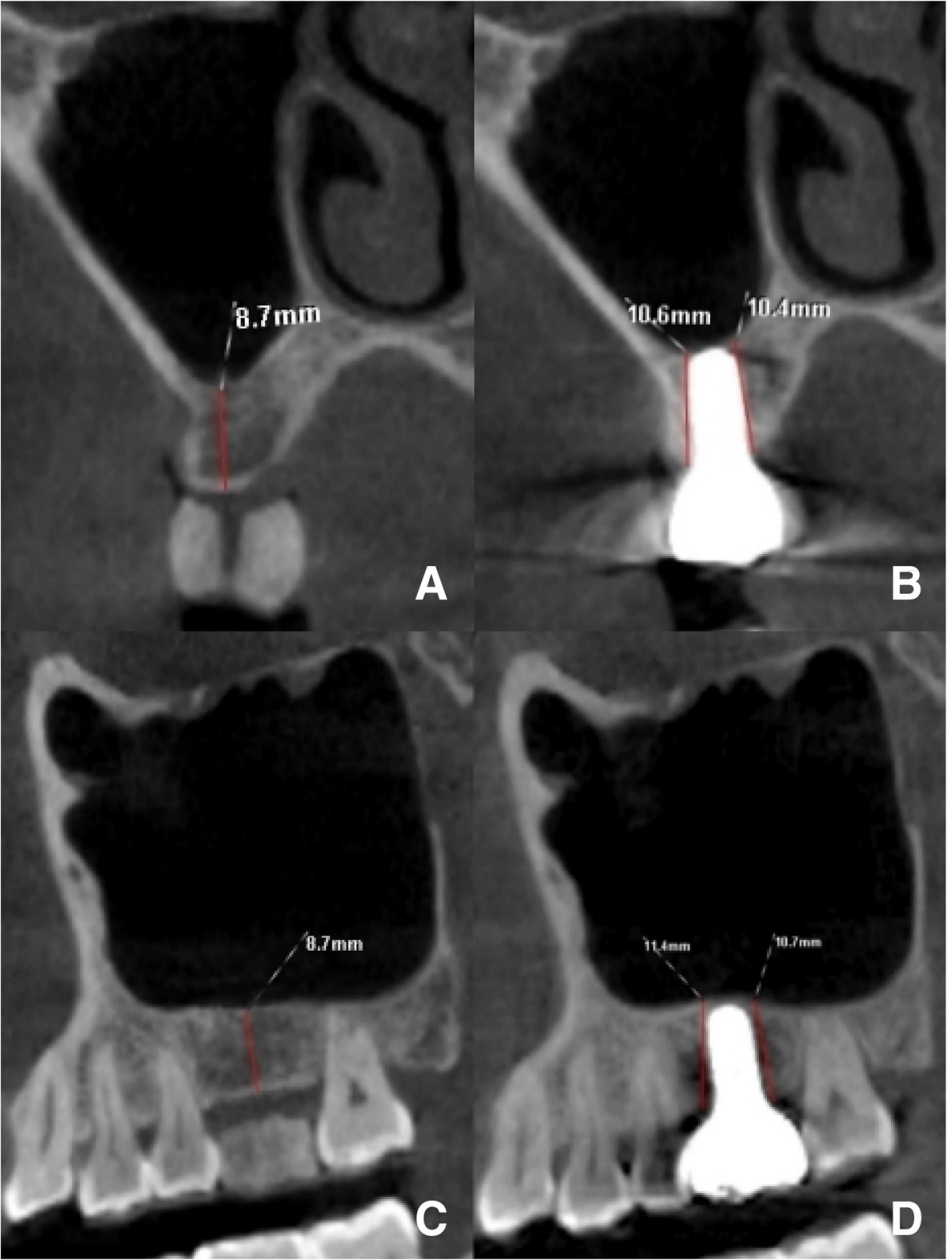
Case 2: The CBCT images of implant site 16 before (left column) and 6 months after surgery (right column). The residual bone height (RBH) was measured from the cortex bone to the alveolar bone crest under the sinus floor in a coronal and c sagittal views. The new bone level was measured from the most coronal implant thread to the most apical visible implant at b buccal and palatal sites in coronal view and d mesial and distal sites in sagittal view
